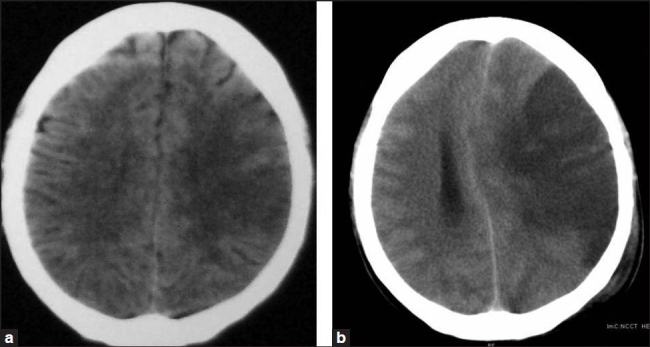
Computed tomography of brain (plain) showed (a) left MCA territory infarction having no midline shift (on admission); (b) large haemorrhagic infarction in the left MCA territory with a gross midline shift after 36 h
